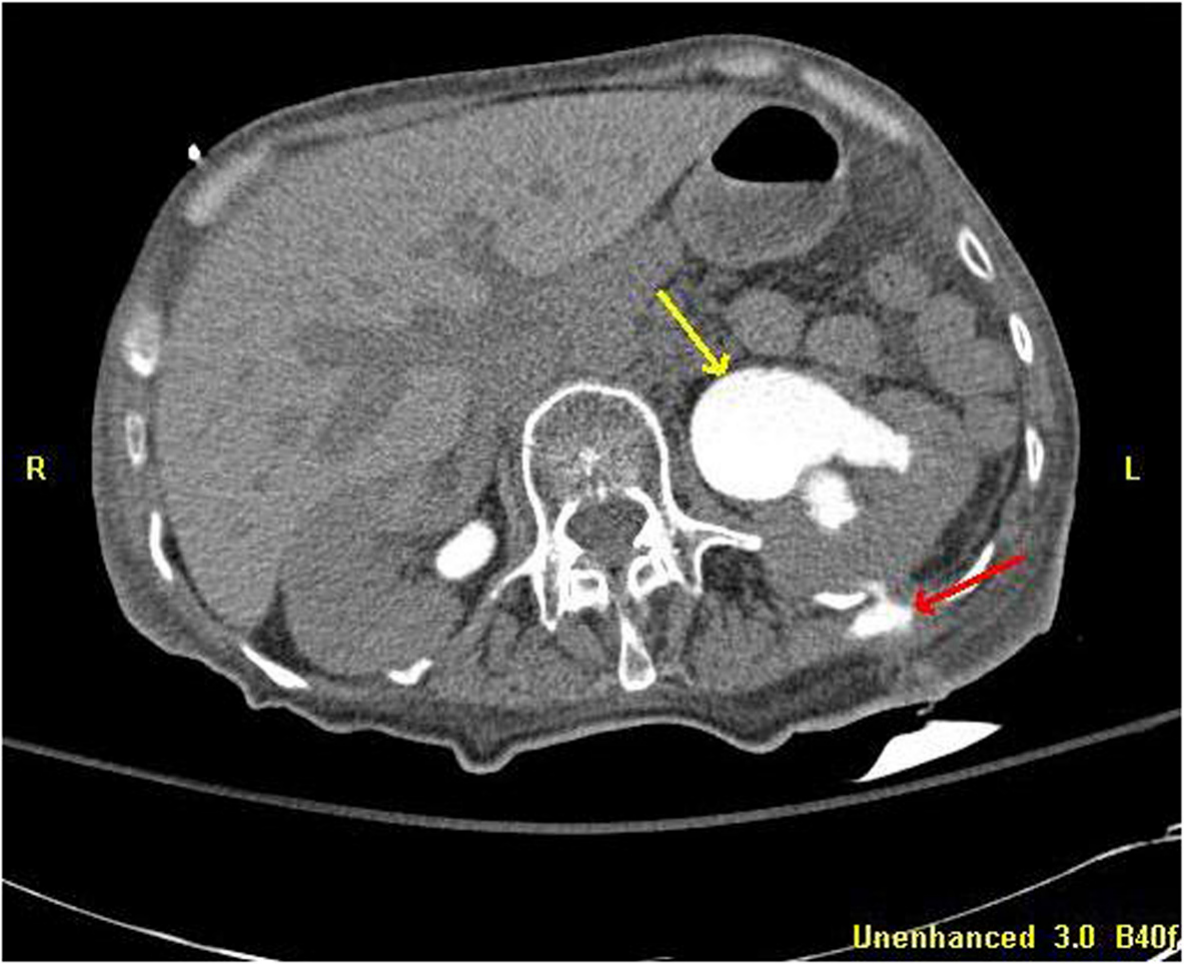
Computed tomography urogram demonstrating contrast extravasation from a calyceal rupture in the mid-left kidney (red arrow) as well as left-sided hydronephrosis (yellow arrow).
